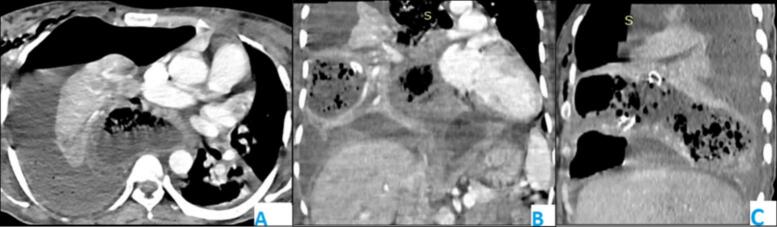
Thoracoabdominal Contrast-Enhanced CT Scan: (A) Axial, (B) Coronal, and (C) Sagittal views demonstrating a diffusely dilated esophagus consistent with achalasia, multifocal consolidation in the right lung, a cavitary lesion with an air-fluid level in the right lower lobe, and a moderate right pleural effusion with a chest tube in situ.
